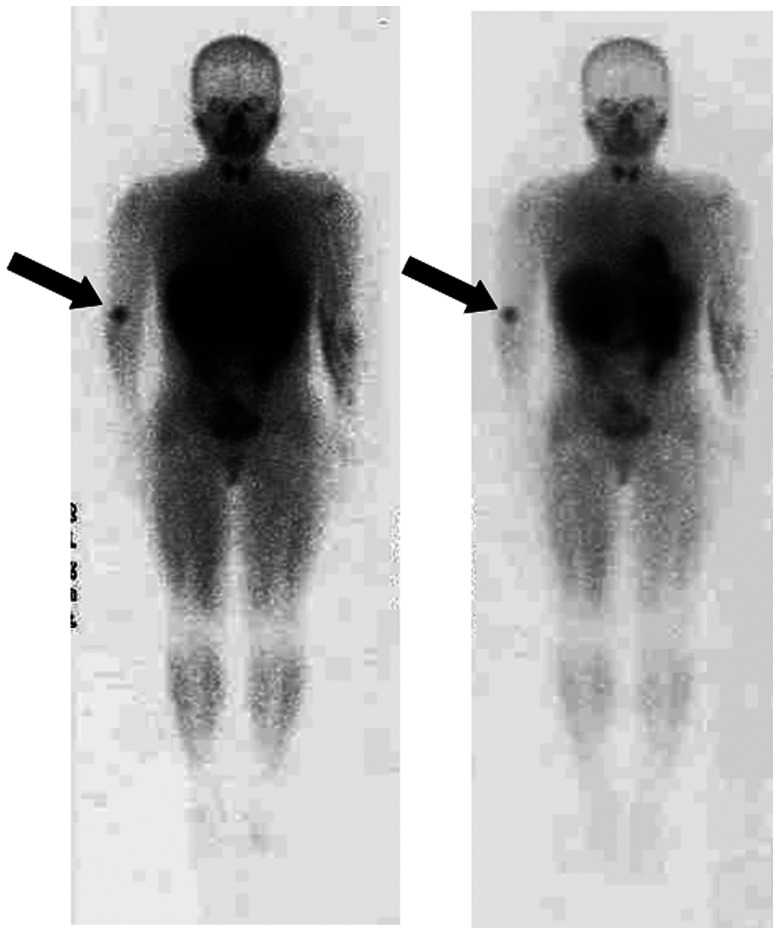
Thallium-201 scintigrams showing an abnormal accumulation (arrows) consistent with the tumor in the early (left) and delayed (right) phases. Such an abnormal accumulation was not observed at any other site.
